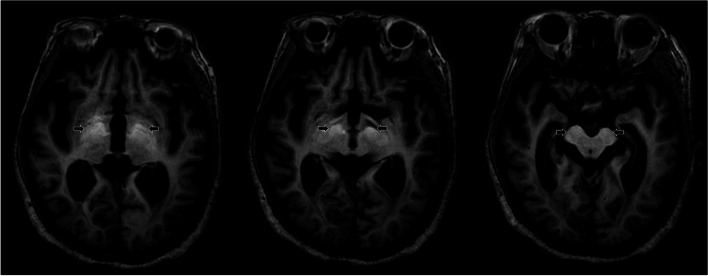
Brain MRI shows bilateral symmetric high signal intensity at the globus pallidus and substantia nigra on T1-weighted image (arrows)
